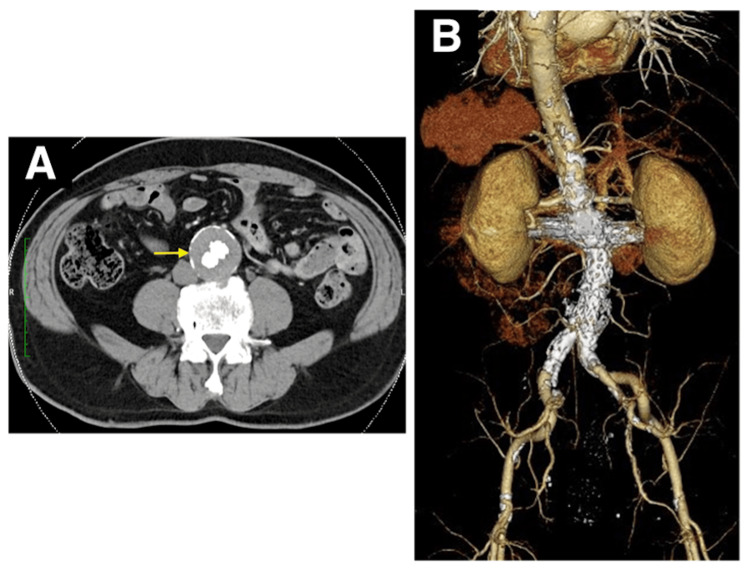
(A and B) Postoperative three-dimensional reconstruction computed tomography and computed tomography angiography showed favorable stent graft positions without type Ia endoleak.
